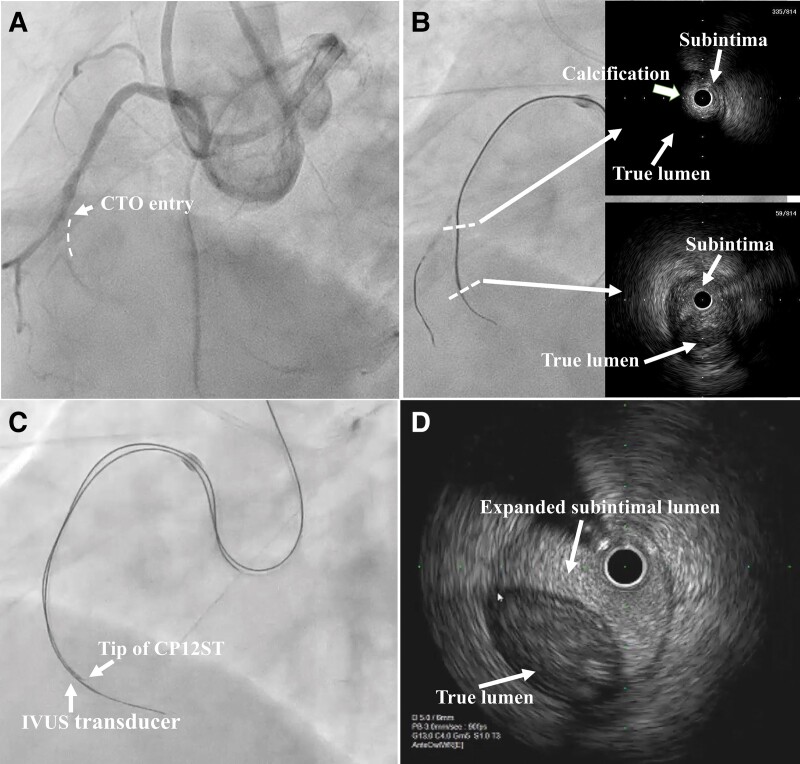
Simultaneous coronary angiogram and intravascular ultrasound assessment in the chronic total occlusion. (*A*) Coronary angiogram. (*B*) Intravascular ultrasound examination from subintimal space. (*C*) Intravascular ultrasound–guided tip detection. (*D*) Intravascular ultrasound image showing subintimal space expansion. CTO, chronic total occlusion; IVUS, intravascular ultrasound; CP12ST, Conquest Pro 12 Sharpened Tip.
